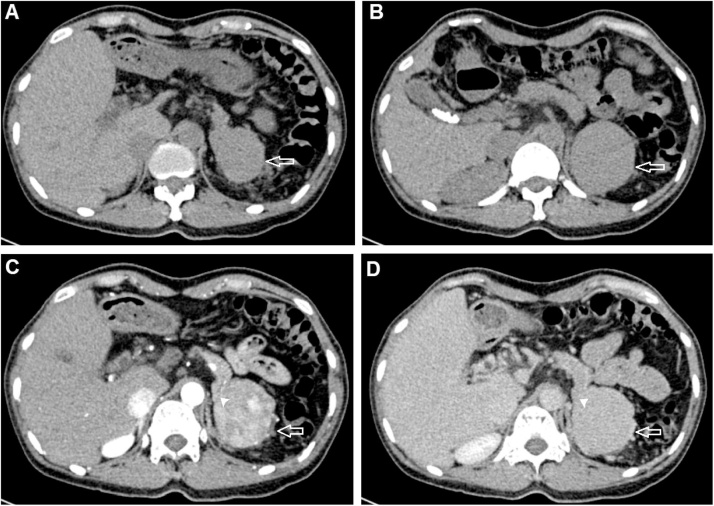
Axial CT scanner precontrast (A and B), in the arterial phase (C), and in the venous phase (D). There was a mass located in normal spleen site exhibiting isodensity with hepatic tissues (A and B, arrow), inhomogeneous enhancement in the arterial phase (C, arrow), and homogeneous enhancement in the venous phase (D, arrow). It was suspected that the mass developed from the pancreas tail (C and D, arrowhead).
